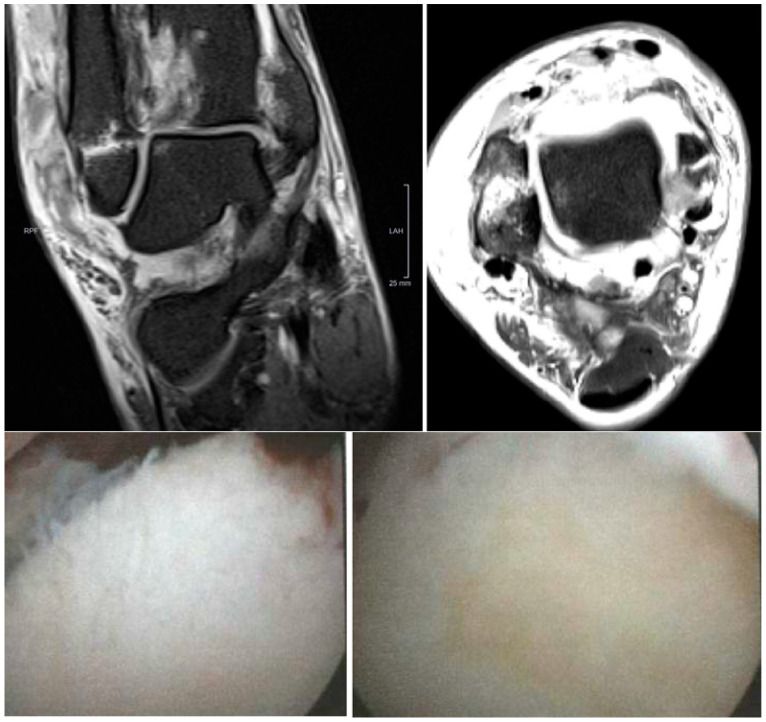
Coronal (above left) and axial (above right) proton-density-weighted turbo-spin-echo fat-saturation MRI sequences (PD tse fs) showing a 4 × 4 mm ICRS 1b CL in zone 5 of the talar dome, with subchondral edema in a 31-year-old male with an acute ankle fracture. Below are the intraoperative figures of the corresponding lesions in arthroscopy in zone 5 of the talar dome measuring 8 × 10 mm and grading ICRS 2.
